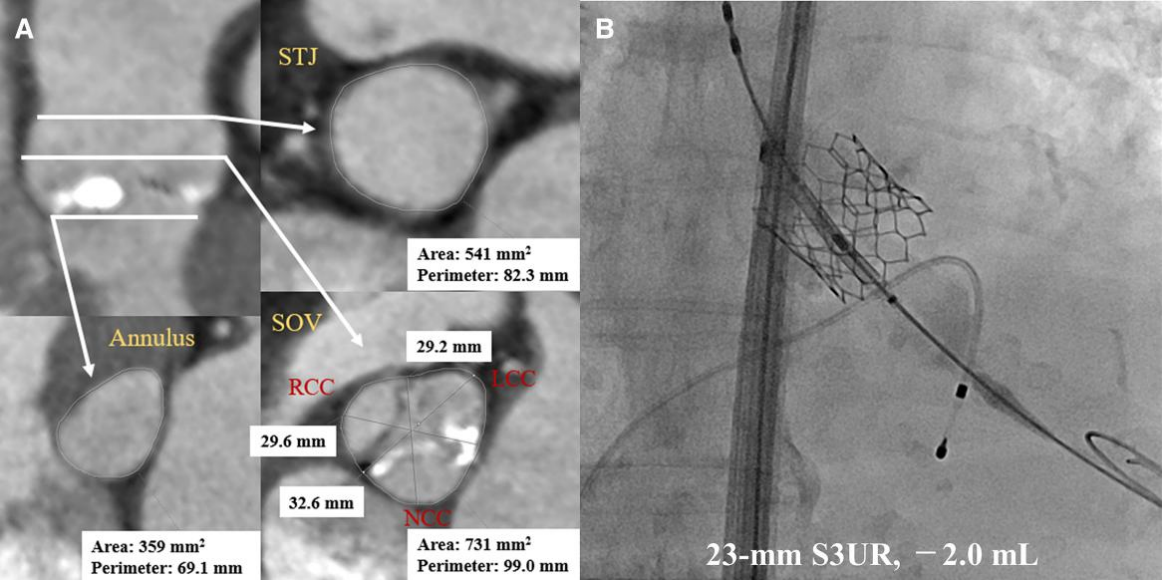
Images of the patient. (*A*) Preprocedural findings on contrast-enhanced computed tomography. Aortic annular area and perimeter are 359 mm^2^ and 69.1 mm, respectively. (*B*) The implanted 23 mm Sapien3 Ultra Resilia transcatheter heart valve with a 2 mL underfilling volume.
